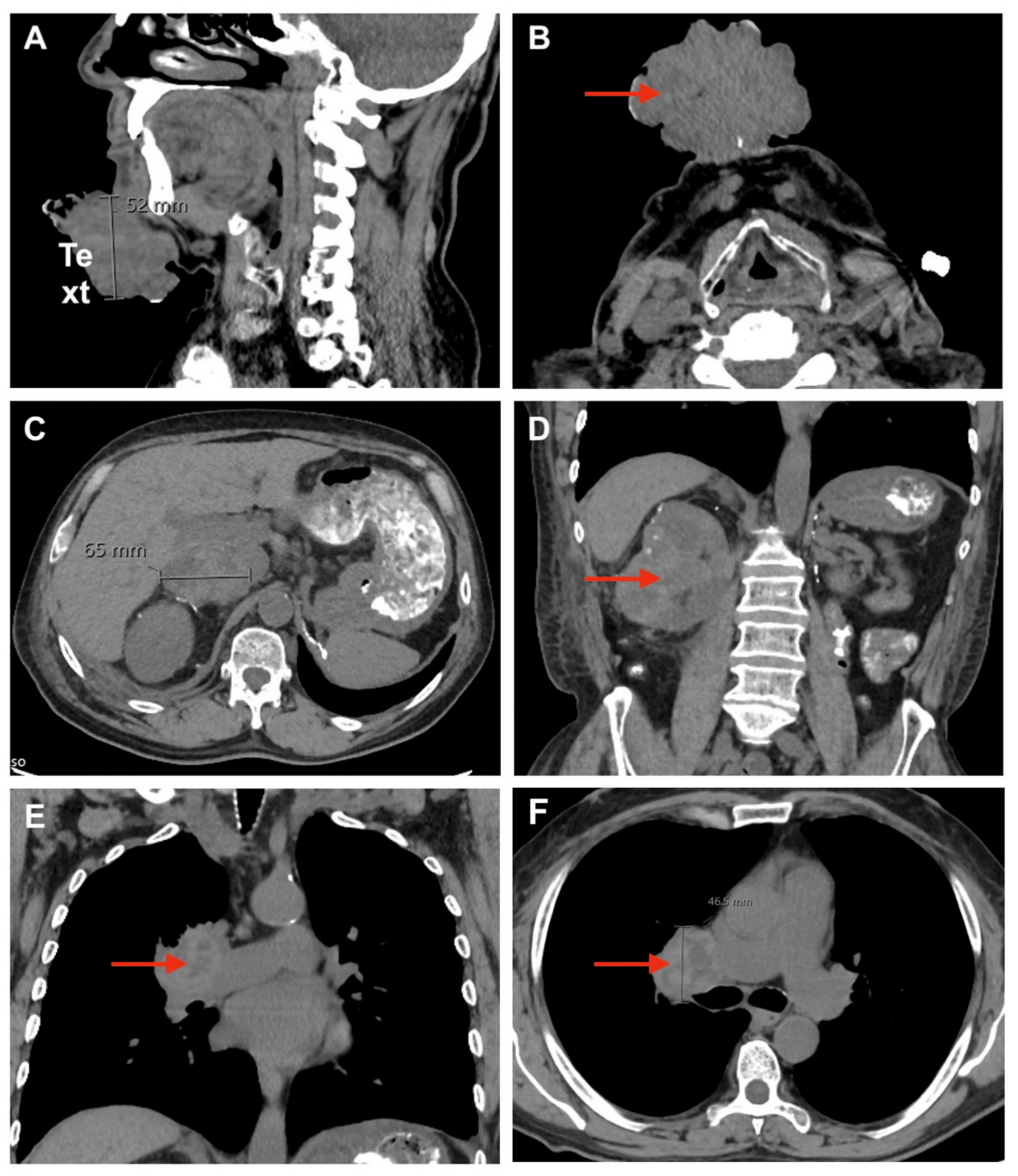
Computed tomography images obtained on admission A: sagittal view of fungating, ulcerated superficial mass arising from the skin overlying the mental protuberance without evidence of deep extension B: axial view of the same mental mass C: axial image demonstrating a grossly abnormal right kidney suspicious for recurrent neoplasm D: coronal view of the same grossly abnormal right kidney E: coronal image of the thorax showing a pulmonary nodule consistent with widespread metastases F: axial image of the thorax showing a pulmonary nodule consistent with widespread metastases
